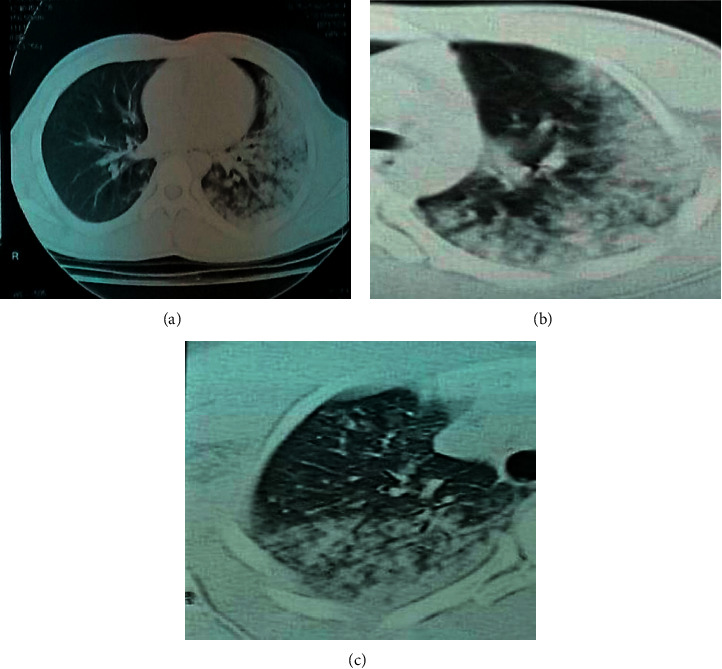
3 CT scan images classifying lung contusions. (a) Total areas on the left lung filled with lesions (score 4); (b) lesions filling the whole lateral and part of medial lung (score 3); (c) contusion not filling all the lateral nor the medial areas (score 2).
